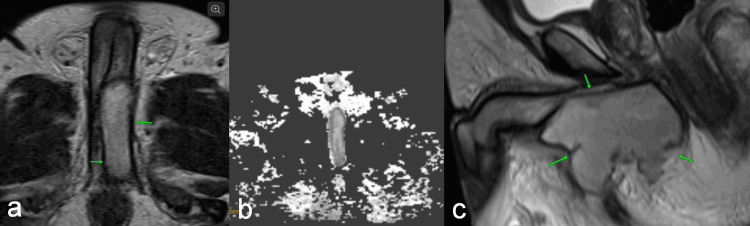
T2 hyperintense image of penis (A), DWI of penis (B) and axial imaging of perineum showing an abscess (C). (A) T2 hyperintense image showing a collection measuring 7.3 x 5.1 × 7.6 cm volume of 132 cc is noted in the left corpora cavernosa, (b) DWI of penis showing abscess extending into corpora cavernosa with no breach into tunica and urethra, and (c) axial imaging of perineum showing abscess collection: Anteriorly extending to the root and penis, extending into the left corpora cavernosa muscle and splaying the urethra and corpora spongiosa. Posteriorly, limited to the perineum with no gross extension into the anal canal. Inferiorly extending into the root of the scrotum and the anterior aspect of the left gluteal cleft. Superiorly seen displacing the left pubococcygeus and puboanalis muscles; however, no extensions beyond it. No extensions into the prostate without tunical or urethral breach. These multiparametric MRI  features represent a left cavernosal abscess with an extension into the perineum. DWI: Diffusion-weighted imaging
